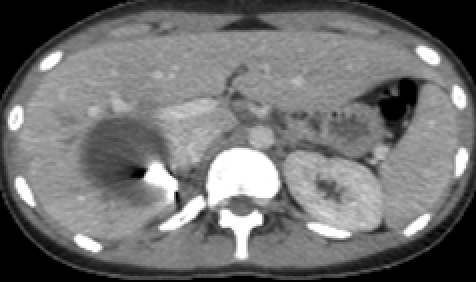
Follow–up CT scan, 10 days after embolization
